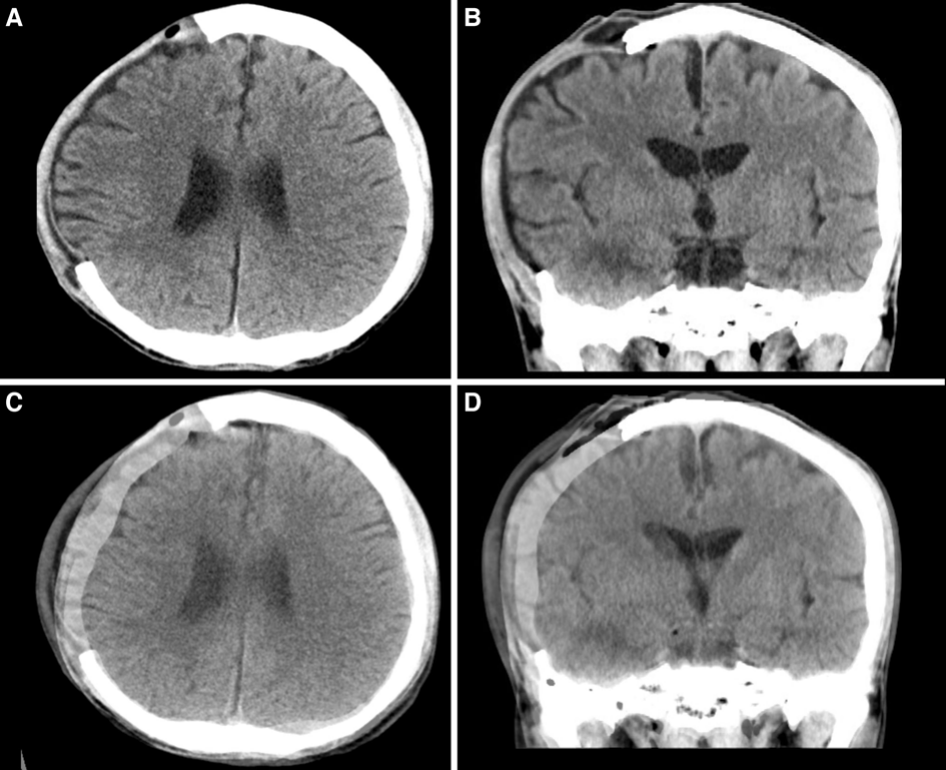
CT of the head. (A) Axial and (B) coronal views of the greatest diameters of skull defect before CP. Presence of interhemispheric hygroma is noted. (C) Axial and (D) coronal views of the pre-CP images adjusted to 50% transparency superimposed onto corresponding pre-DC images (Fig. 1). All brain matter lies within the outer table of the skull. CP, cranioplasty; CT, computed tomography; DC, decompressive craniectomy.
